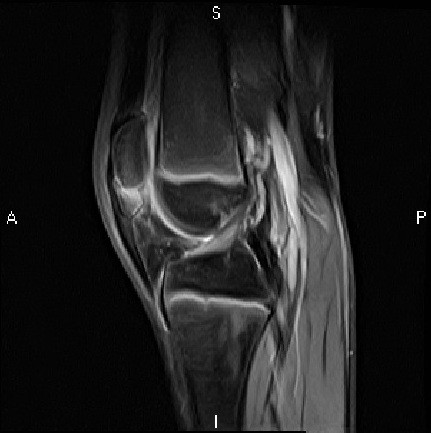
T2-weighted sagittal MRI of Case 1 at 2 weeks.
